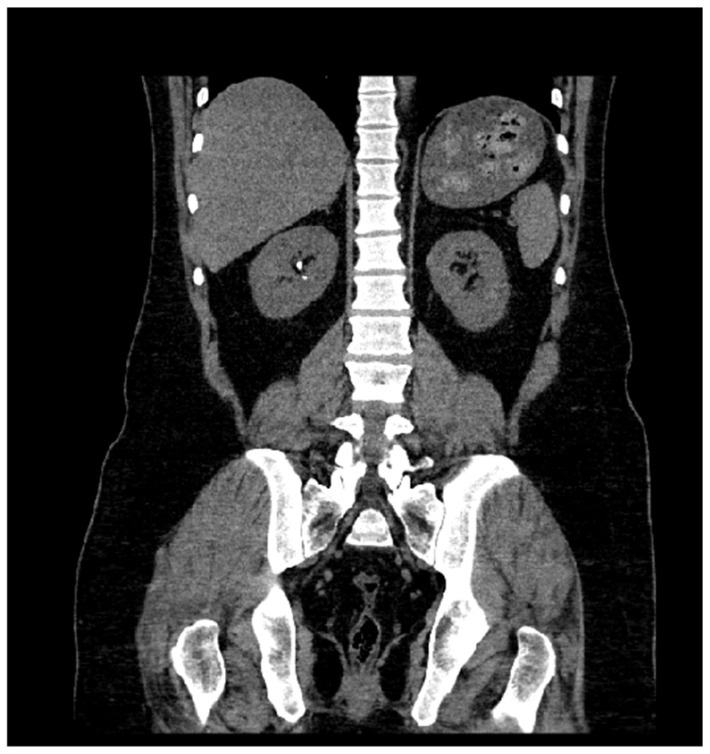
Coronal CT KUB image taken from the PACS database. The right kidney shows several visible hyperdense calcifications, consistent with renal stones. The image also highlights overscanning above the upper poles of the kidneys, which unnecessarily exposes additional tissue to radiation.
